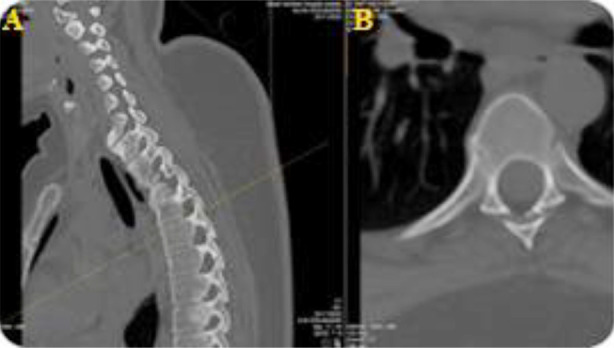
CT scan image demonstrating an Anderson lesion in a patient diagnosed with ankylosing spondylitis. A: Sagittal plane, B: Axial plane
